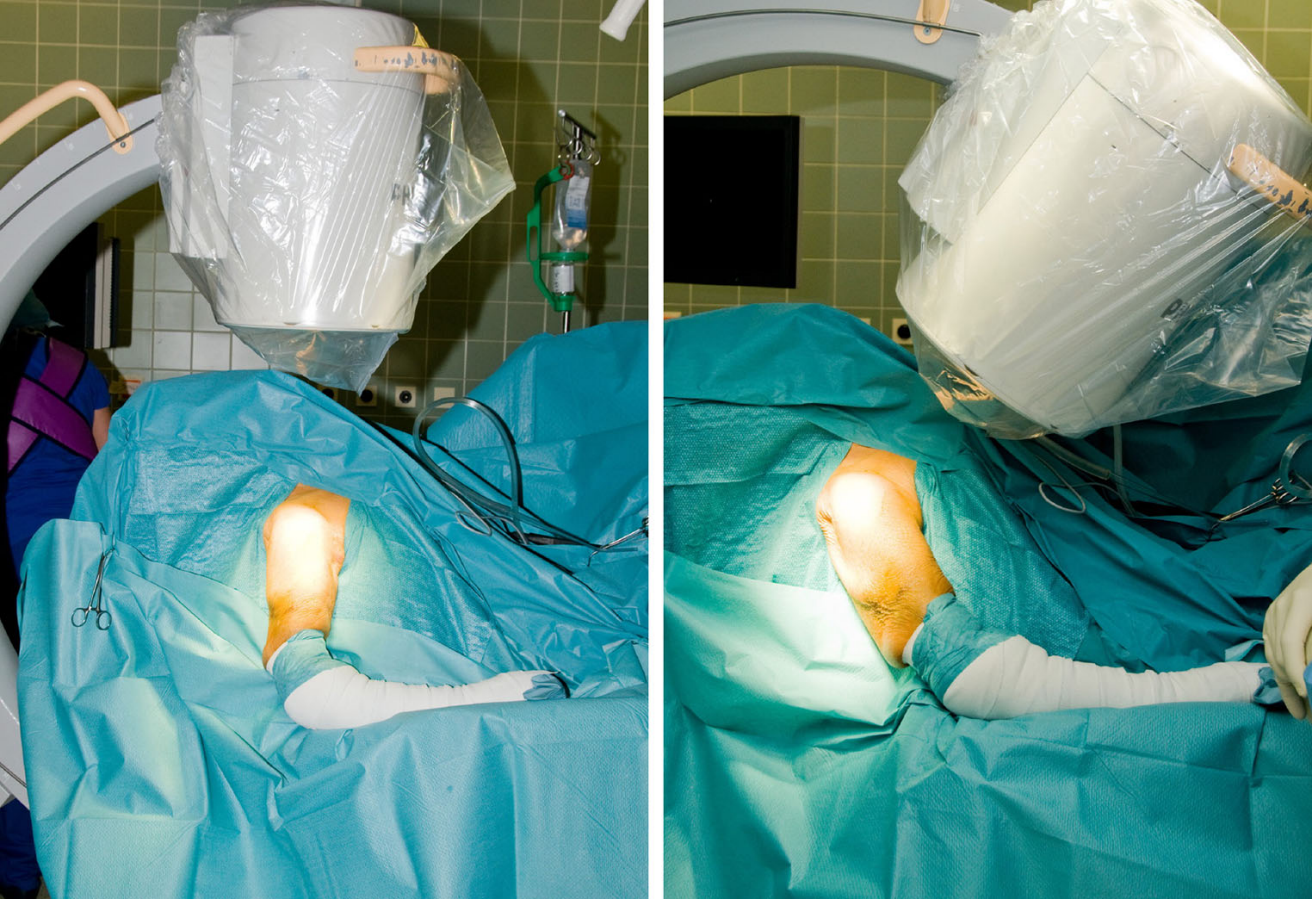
Position of X-ray tube for screening during LBN insertion
